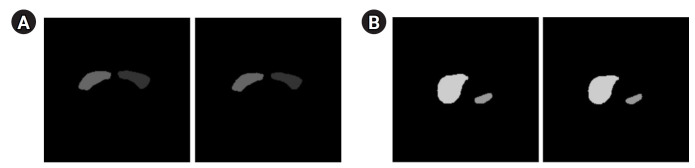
Results of positron emission tomography/computed tomography alignment using the MIM program for breast (A), and liver and spleen (B). In each image, the left side displays results from the MIM program, while the right side shows results from our technique.
